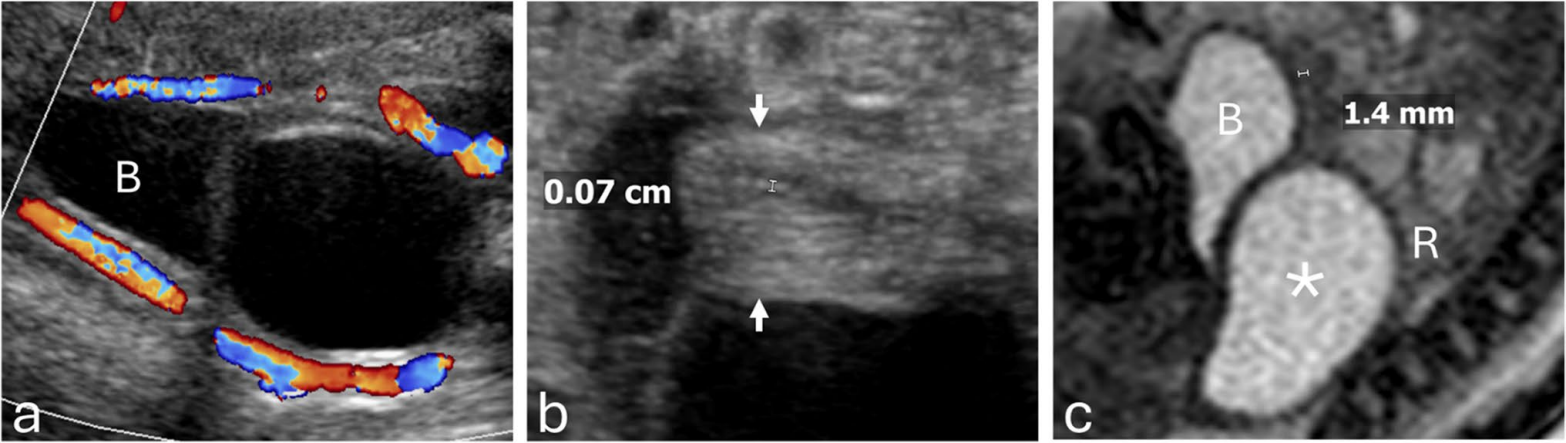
a Prenatal grayscale ultrasound with color Doppler at 33 weeks shows imperforate hymen with hydrocolpos. The fluid-distended vagina (asterisk) appears cystic, posterior and slightly inferior to the bladder (B) and anterior to the rectosigmoid colon (R). b Sagittal grayscale ultrasound shows the uterus (arrows) with a non-thickened endometrium measuring < 2 mm. c Sagittal T2-weighted MR image confirms hydrocolpos (asterisk) and a non-thickened endometrium. The infant underwent postnatal hymenectomy
